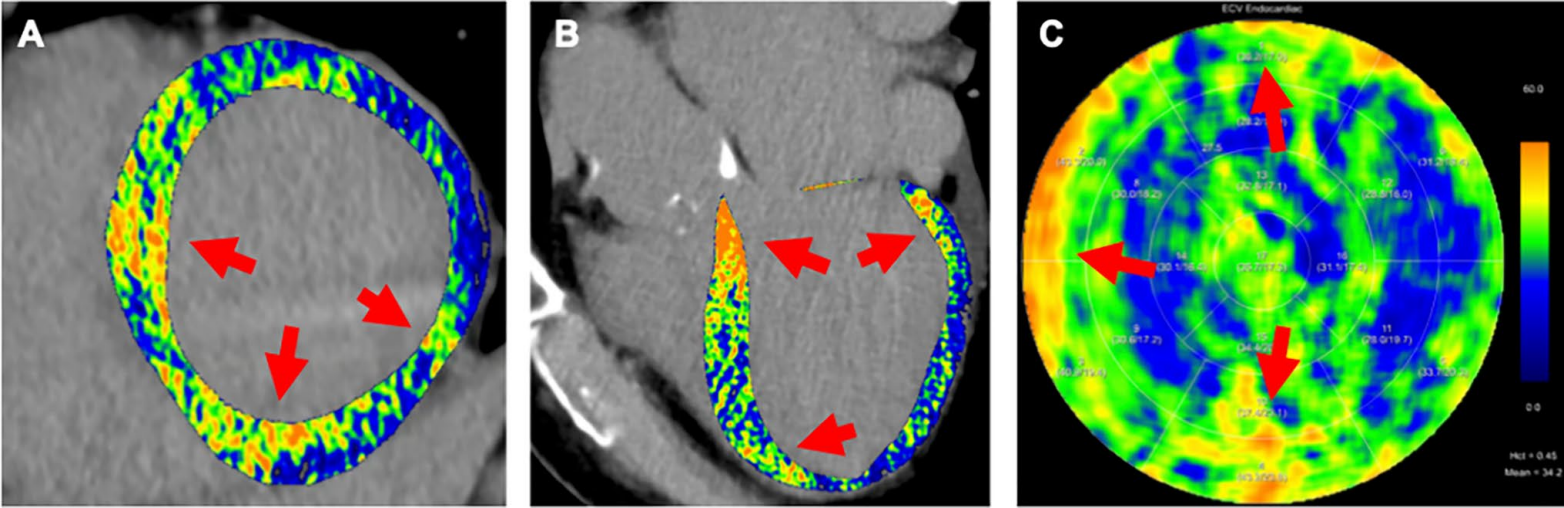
Seventy-five-year-old female patient with confirmed cardiac transthyretin amyloidosis. CT-derived ECV maps are overlaid on late enhancement CT images in A short-axis and B three-chamber views and are represented in the C polar map. Red arrows highlight areas with pronounced myocardial injury. The mean myocardial ECV was elevated at 31.74% (normal: 20–26%)
